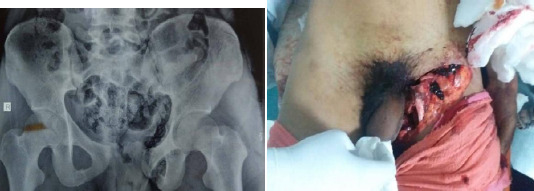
A the time of presentation (A) X-ray anteroposterior view showing pelvic fracture APC Type III (B) Laceration over Left groin.
